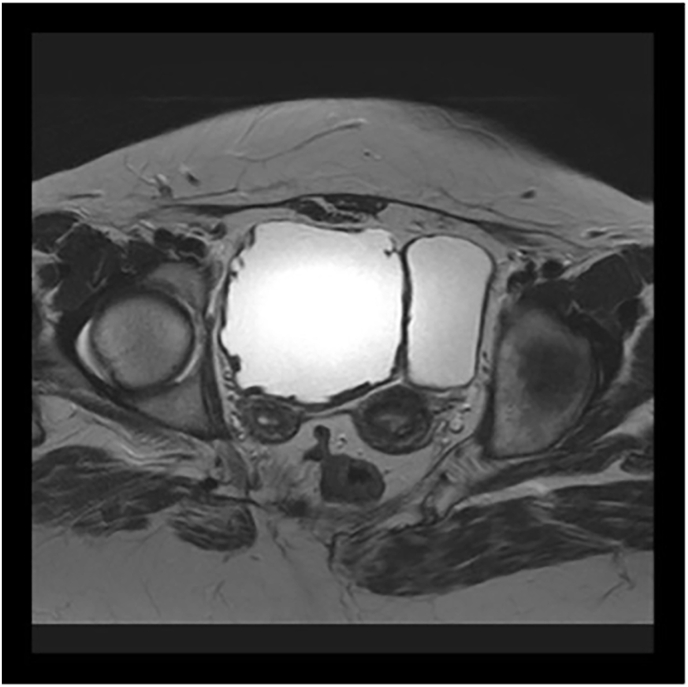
Magnetic resonance imaging, axial plane. Uterus didelphys and duplicated bladder.
